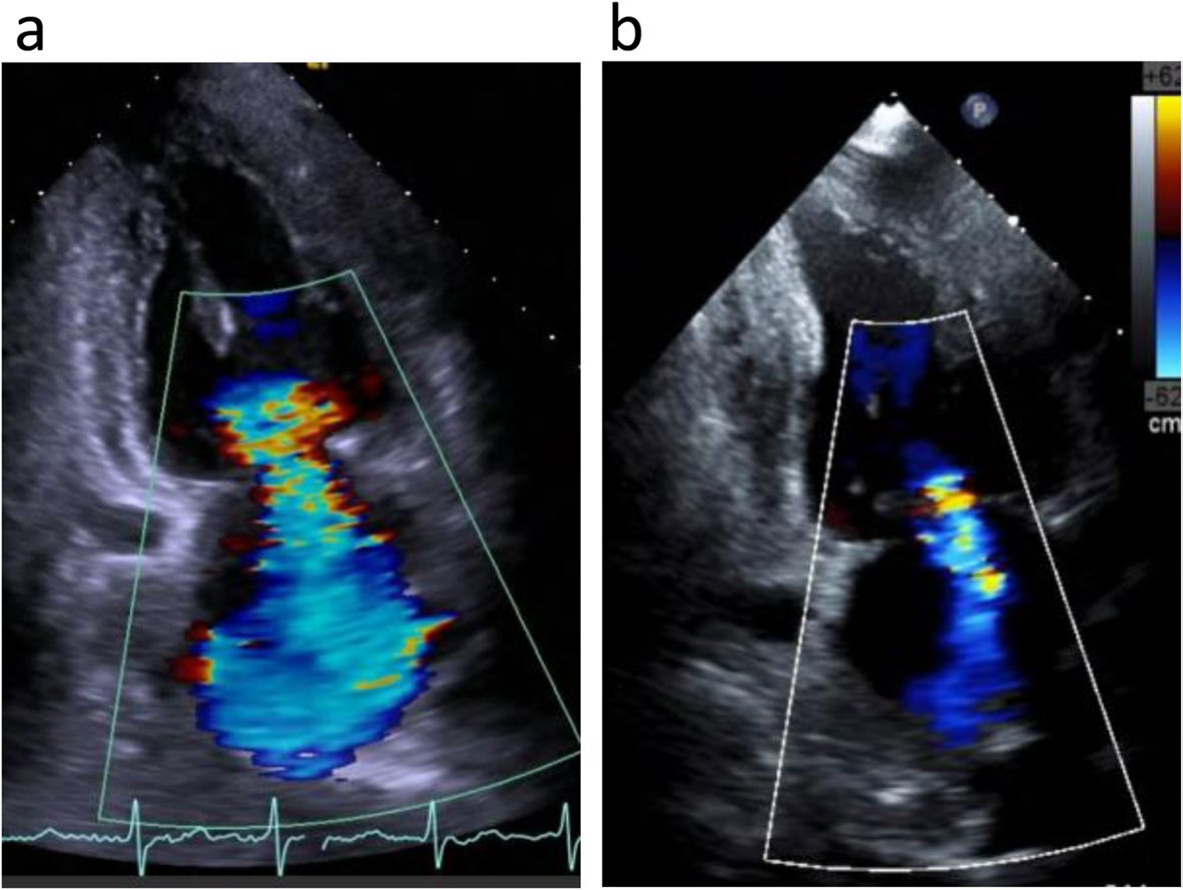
Changes in cardiac function before (a) and after (b) living-donor renal transplantation by transthoracic echocardiography. Left ventricular ejection fraction, left ventricular end-diastolic diameter, and left ventricular end-systolic diameter were 30%, 57 mm, and 50 mm at the preoperative point (a) and 50%, 50 mm, and 38 mm at postoperative day 180 (b), respectively. Severity of mitral regurgitation, effective regurgitant orifice area (proximal isovelocity surface area), and tricuspid regurgitant pressure gradient were severe, 0.25 cm^2^, and 30 mmHg at the preoperative point (a) and mild, 0.12 cm^2^, and 21 mmHg at the postoperative day 180 (b), respectively. The above results show that the patient’s cardiac function and severe mitral regurgitation improved to a degree that indicated that cardiac surgery was no longer necessary
